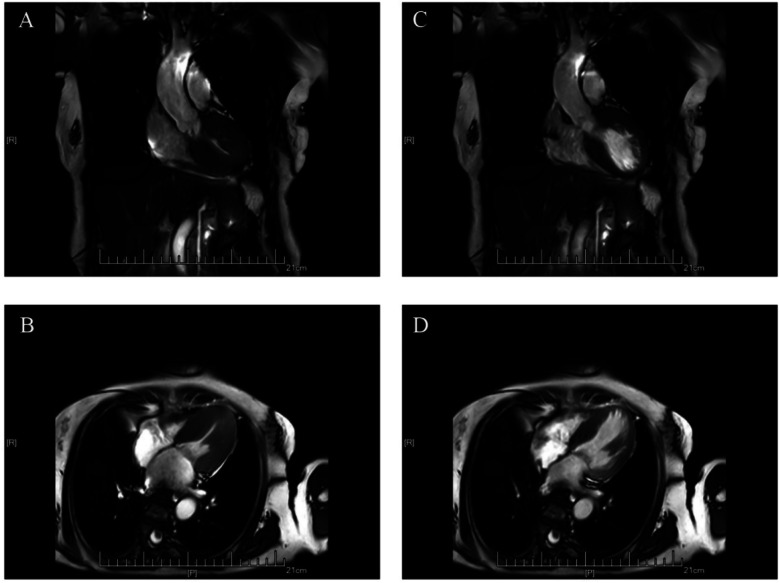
Cardiac MRI scans showing hypertrophic cardiomyopathy. (A,C) Coronal views; (B,D) Axial views.
